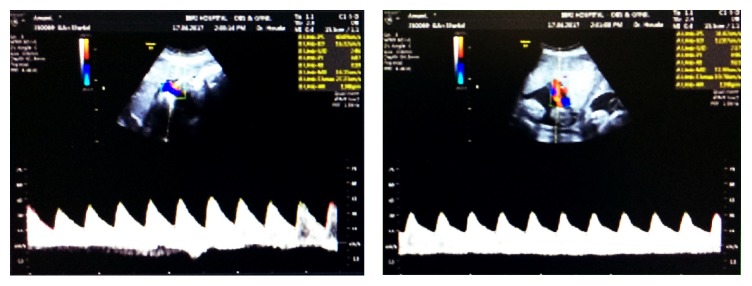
Umbilical artery Doppler for both fetuses at 33 weeks of gestation.
